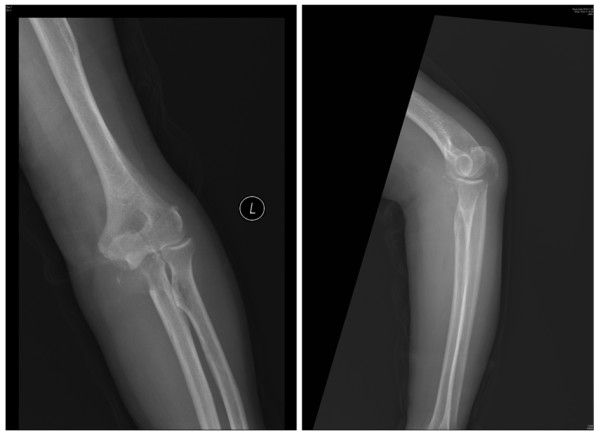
Preoperative radiographic and computer tomographic (CT) images clearly indicate previously diagnosed and current lung metastatic tissues.
